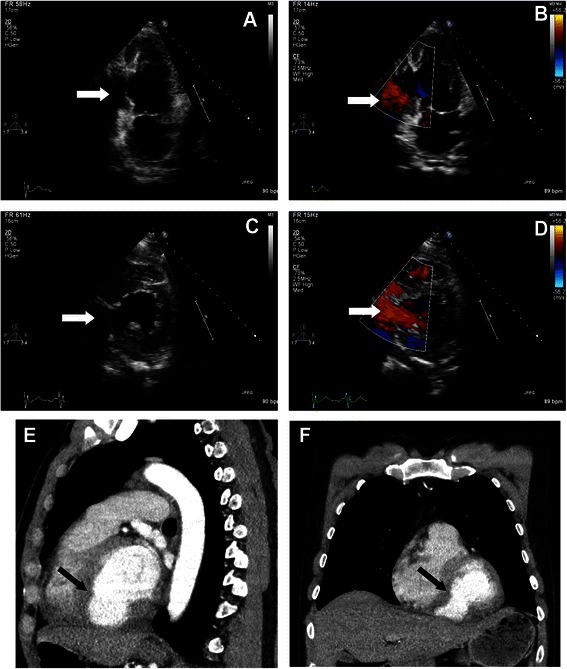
Preoperative TTE and CT. A preoperative transthoracic echocardiogram showing the left ventricle and the posteroinferior cavity of the pseudoaneurysm (a), with turbulent flow inside (b). A TTE cross-sectional view showing the large ostium to the cavity (c) and (d). A preoperative CT exam determines that the large posteroinferior cavity extends beneath the septum (e) and (f). The white (A–D) and black (E and F) point to the pseudoaneurysm
